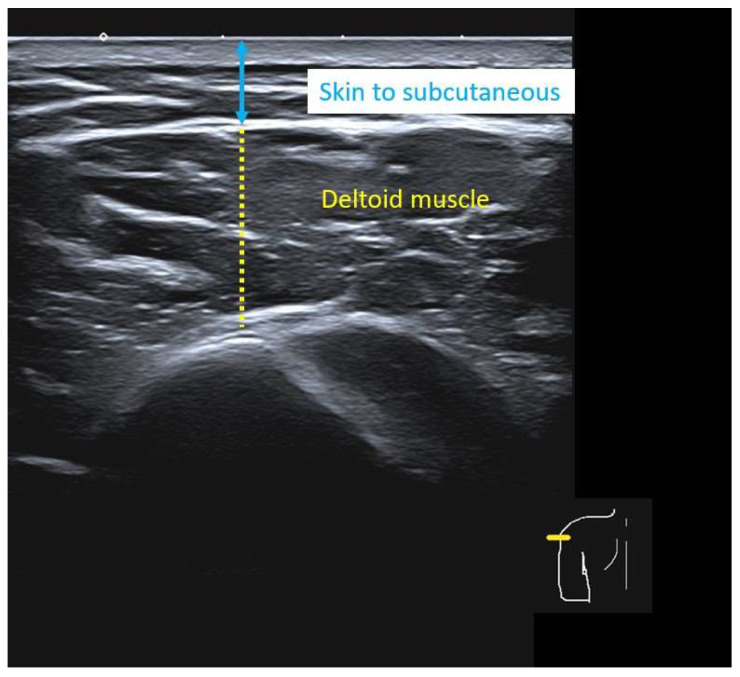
Ultrasonographic image showing the thickness from the skin surface to the deep border of the subcutaneous fat pad (blue double arrowhead) and the deltoid muscle thickness from this border to the subdeltoid fascia (yellow dotted line).
